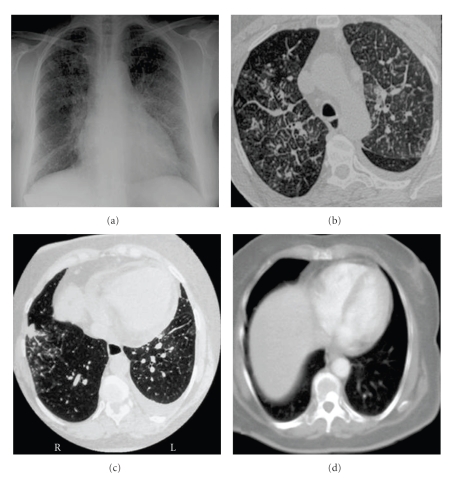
(a) Chest X-ray with interstitial pattern; (b) thoracic high-resolution CT with septal thickening and small left pleural effusion; (c) small nodule in RLL; (d) disappearance of pleural effusion and appearance a vertebral metastasis after 6 cycles of chemotherapy.
